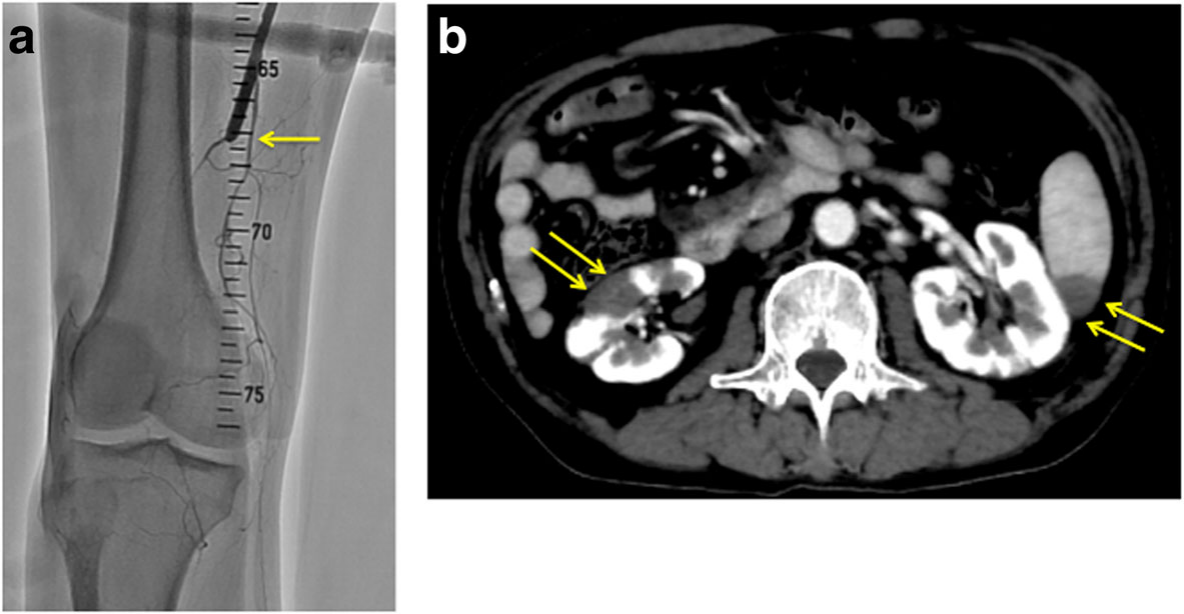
a Angiography revealed obstruction of right distal superficial femoral artery. b Abdominal contrast-enhanced CT scan showed partial infarction of right kidney and spleen
